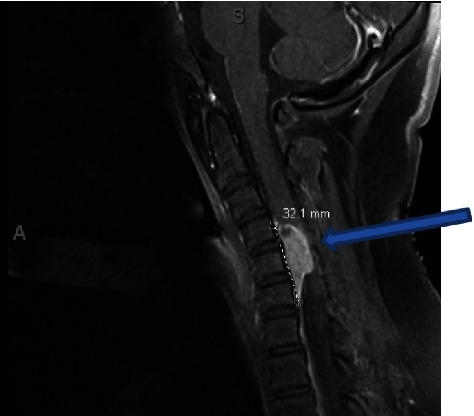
Sagittal T1-weighted preoperative MRI with contrast showing an anterior intradural extramedullary mass, measuring 1.6 × 0.9 × 2.8 cm (blue arrow). The mass is centered at C5 and spans from C4-6 with what appears to be a dural tail. The mass shows significant compression of the spinal cord.
